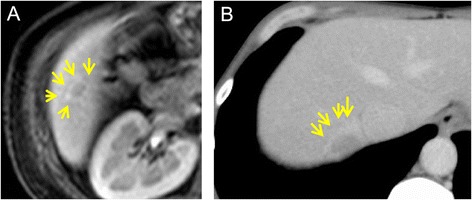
Contrast MRI (A) and CT (B) showed perinodular enhancement in the equilibrium phase.
